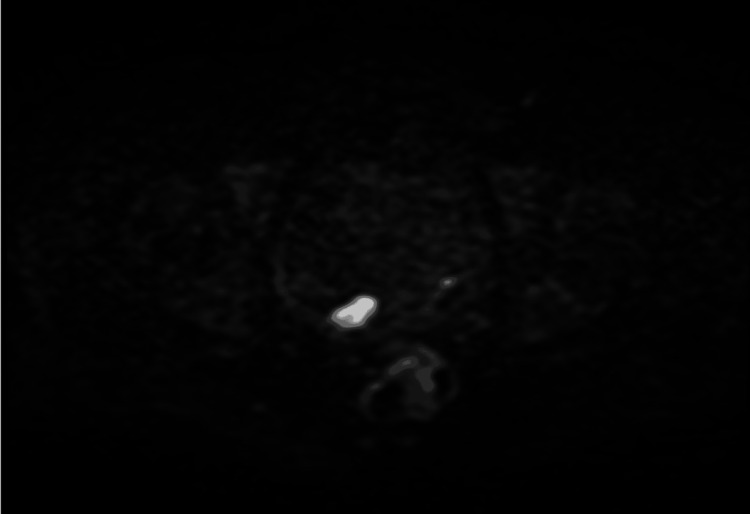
DWI (b = 1000 s/mm²) shows a tumor with hyperintense signal intensity DWI, Diffusion-Weighted Imaging
